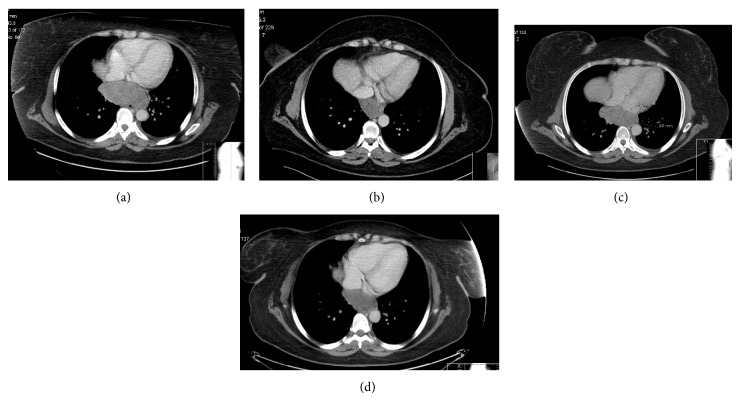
(a) CT scan at diagnosis, Longest Axial Diameter (LAD) 99 mm; (b) CT scan after 10 months of imatinib therapy, partial response LAD 54 mm; (c) CT scan after 13 months of imatinib therapy, disease progression LAD 90 mm; and (d) CT scan after 26 months of imatinib therapy, partial response LAD 44 mm.
